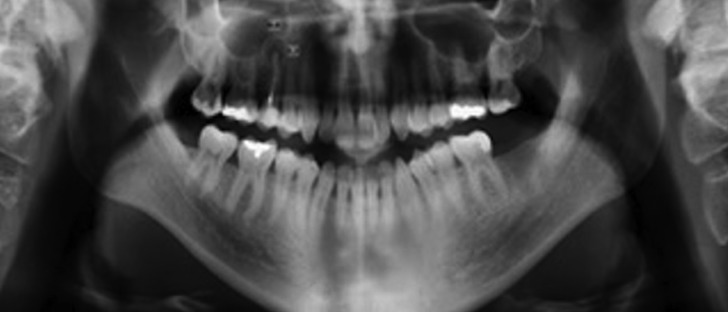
Panoramic radiograph with the measurements made on the radiolucent area of tooth 15.
